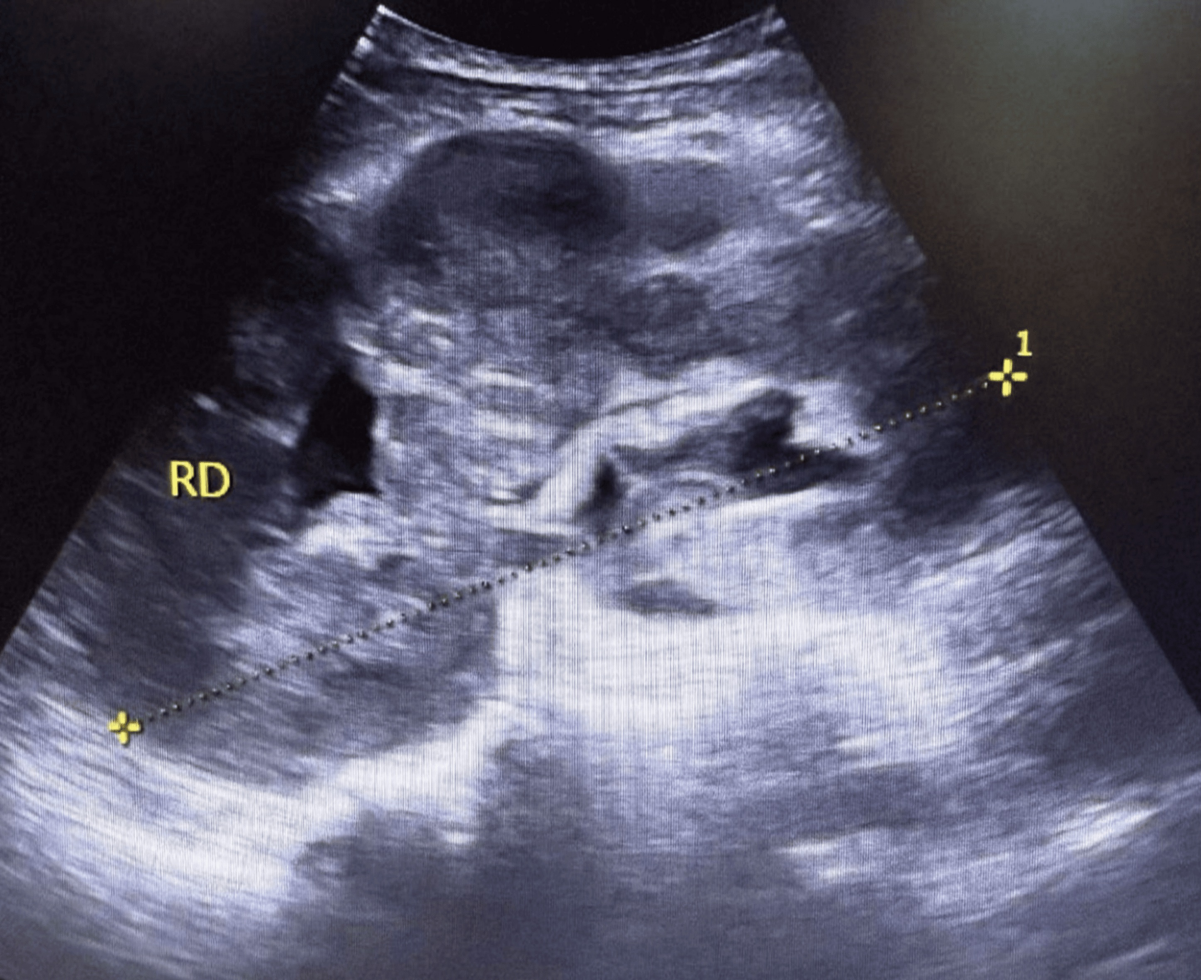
Ultrasound of the right kidney demonstrating renal enlargement with poor corticomedullary differentiation, without evidence of calculi.
